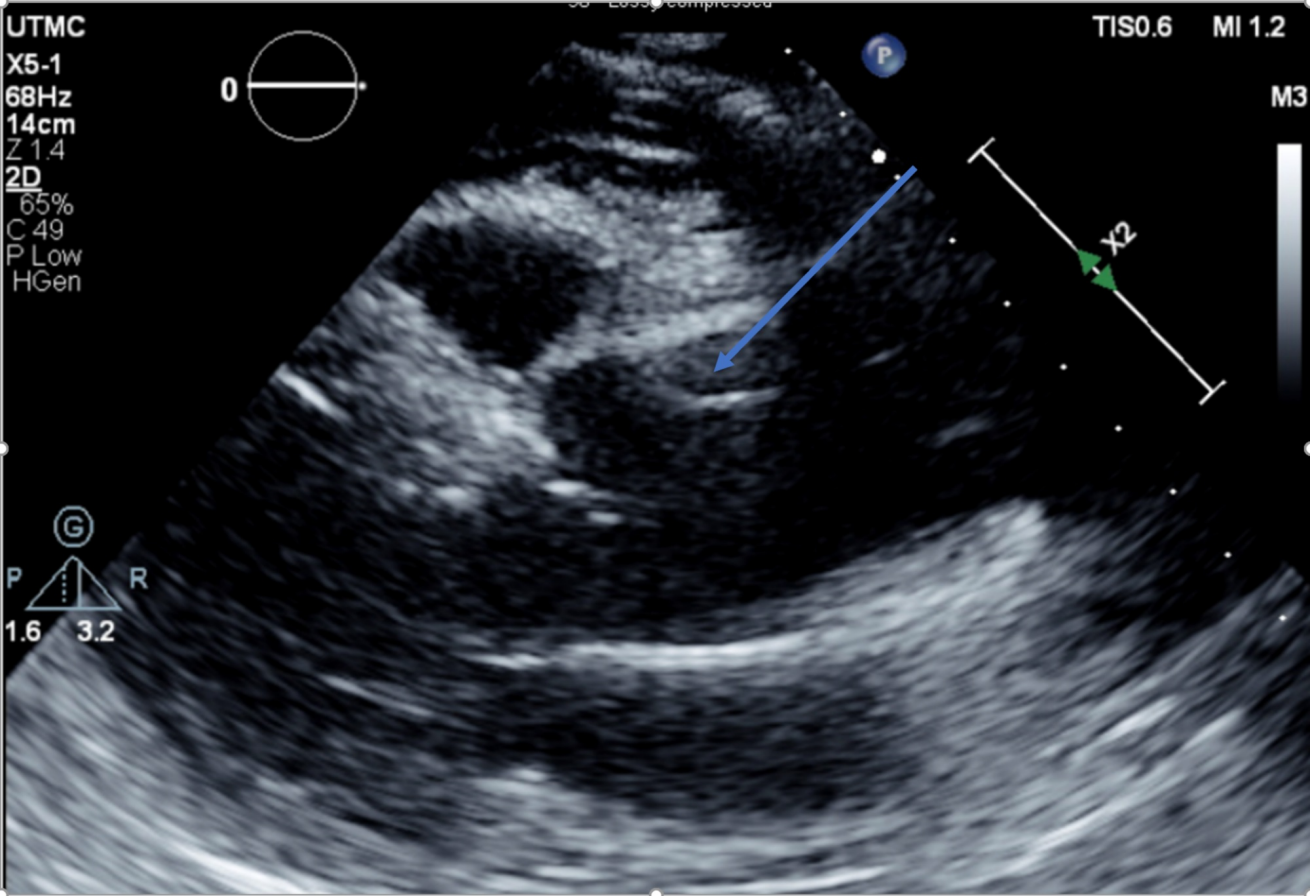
Transthoracic echocardiogram picture showing aortic flap
